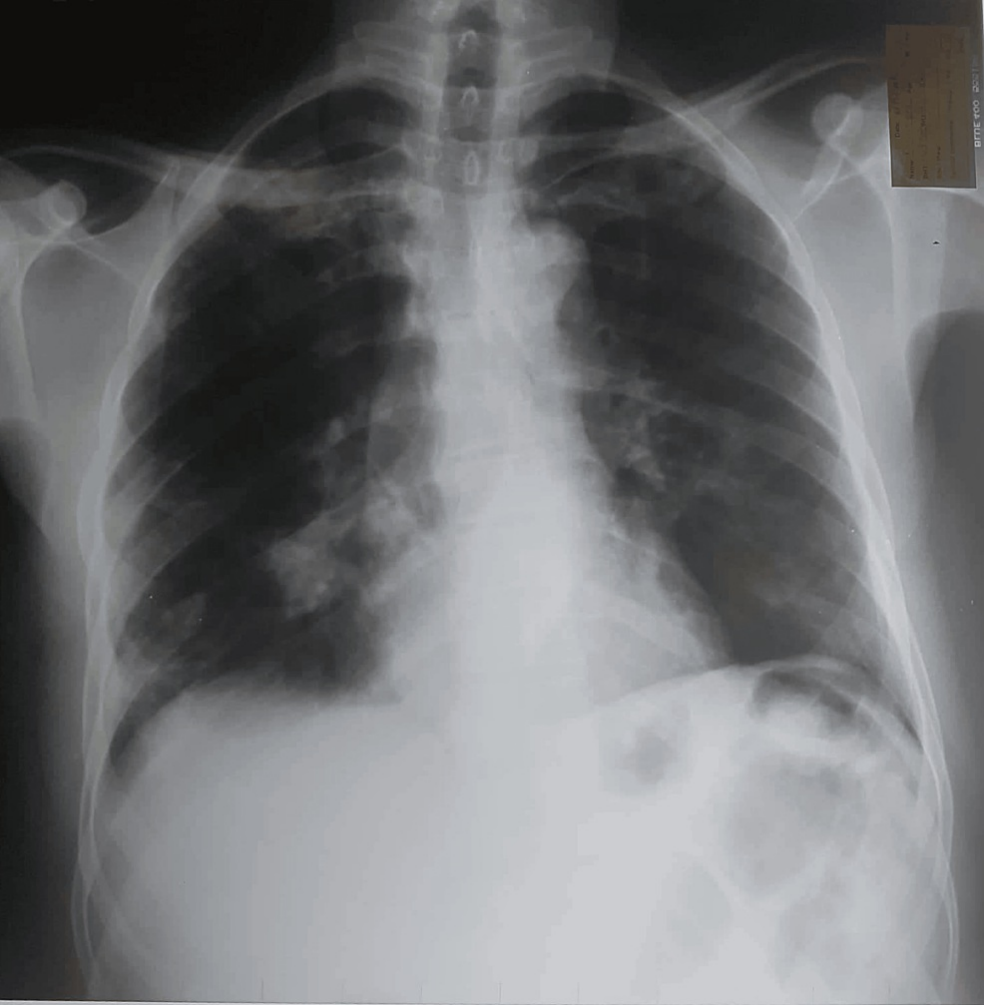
Chest x-ray showing transient pulmonary opacities in bilateral lung bases.
